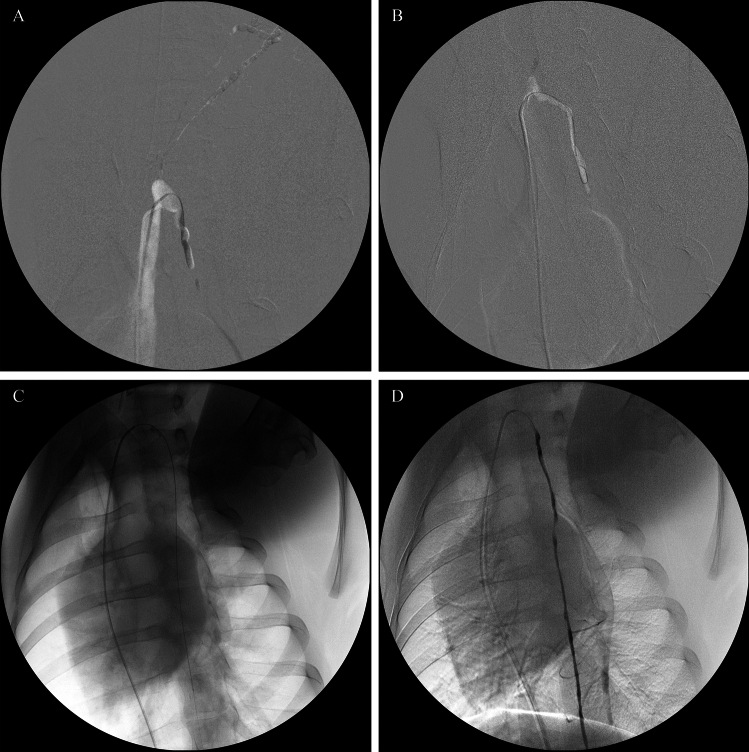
Fluoroscopic images demonstrating procedural manipulations during retrograde cannulation of the thoracic duct (TD) with an X-ray projection of LAO-30°. A Under roadmap guidance, a 0.016-inch, 45° angled GT microwire (Terumo) is advanced from a 5 Fr angiographic catheter through the TD genu into the thoracic part of the TD. B A 0.014-inch straight microwire (NeuroScout, Cordis) is used to navigate a lymphatic valve within the TD; note the bending of the floppy tip facilitating passage. C A microcatheter and microwire assembly is advanced to navigate into the distal TD. D Thoracic ductogram performed via the successfully placed microcatheter, opacifying the TD
